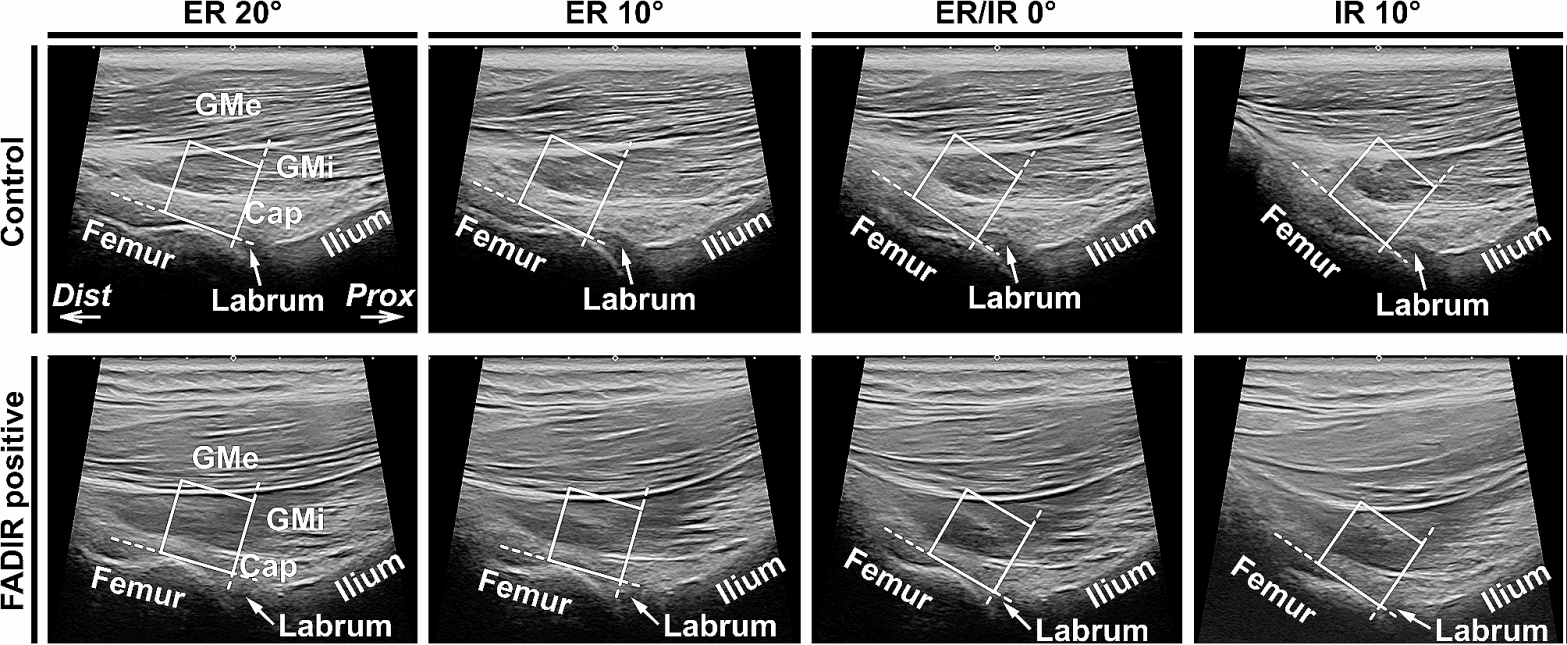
Ultrasound images of the gluteus minimus and joint capsule under rotation in hip flexion. Long axis images of the hip in the coronal plane posterior to the anterior inferior iliac spine. The upper row indicates images of the control group and the lower row, flexion adduction internal rotation (FADIR)-positive group. Images at 20° and 10° external rotations (ER 20° and 10°), 0° external/internal rotation (ER/IR 0°), and 10° internal rotation (IR 10°) in 90° hip flexion are shown from left to right. Squares indicate the regions of interest for orientation measurement
